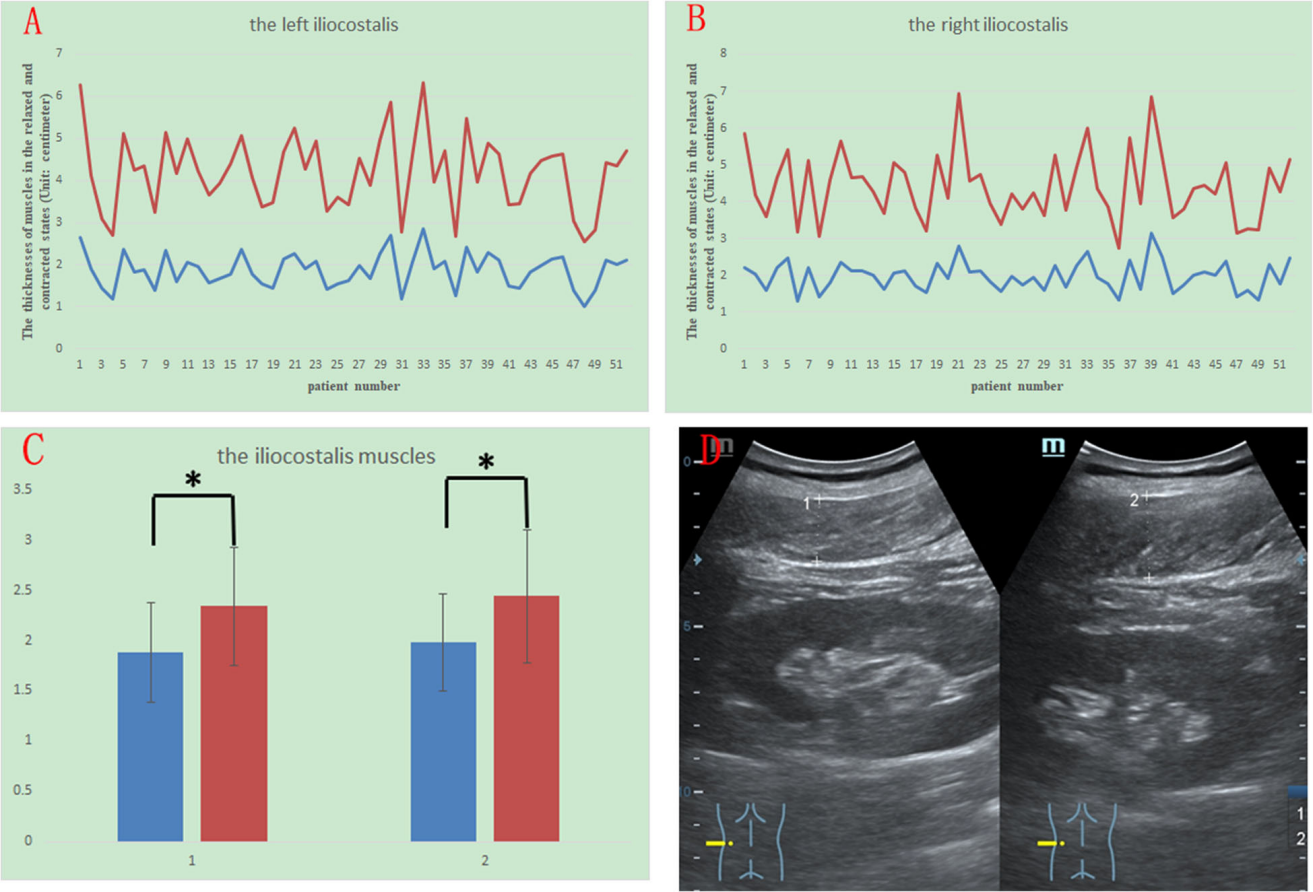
The thicknesses of the left (Fig. 5 A) and right (Fig. 5B) iliocostalis muscles in the resting (blue line) and contracted conditions (red line). Comparison of the thicknesses in the resting (blue column) and contracted conditions (red column). Group 1 is right, and group 2 is left (Fig. 5 C). The sonogram of the iliocostalis: left shows the relaxed state, and right shows the contracted state (Fig. 5D)
